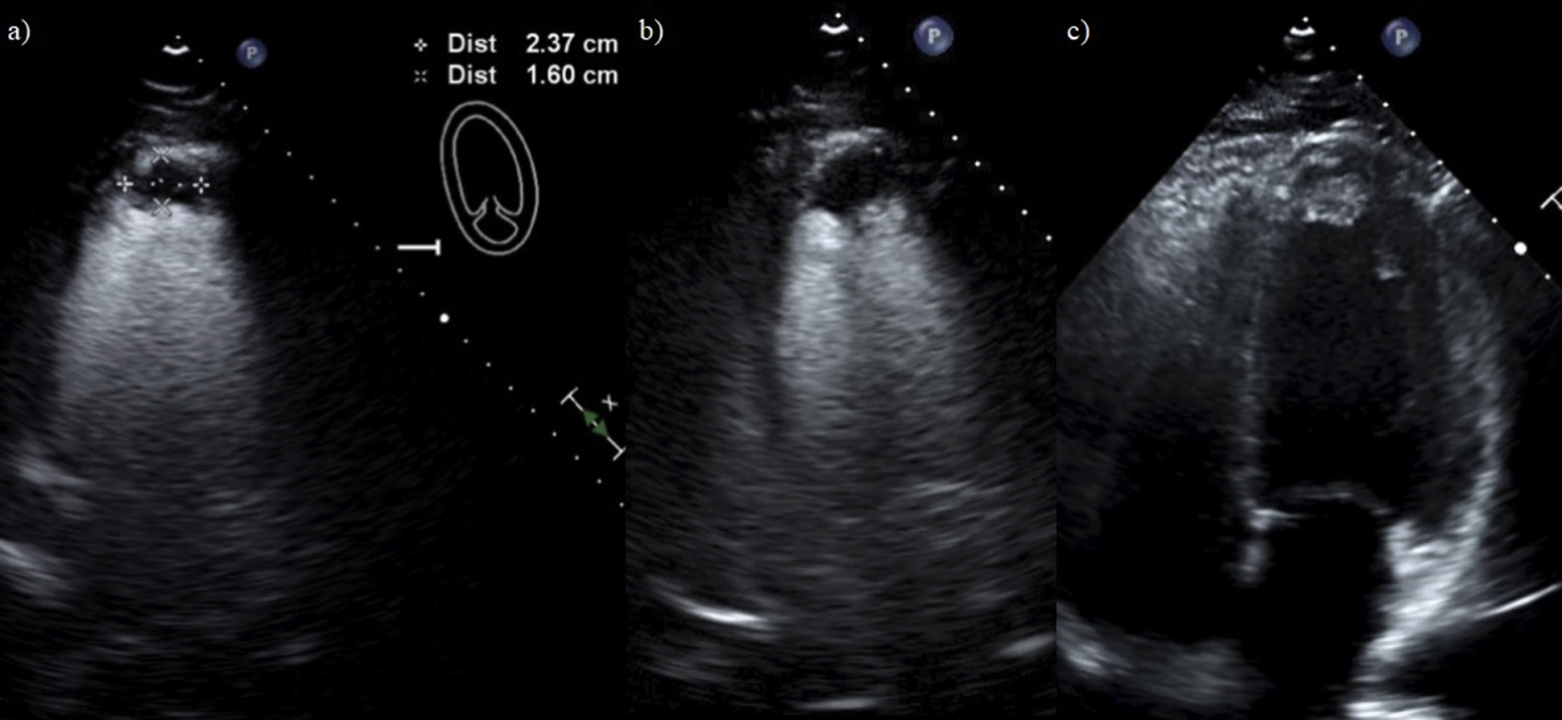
Five-month follow-up transthoracic echocardiogram with and without perflutren lipid microsphere contrast. (A) Perflutren lipid microsphere contrast-enhanced apical two-chamber view of the LV apical mass on follow-up imaging 5 months from the initial images demonstrating reduced size of the mass. (B) Perflutren lipid microsphere contrast-enhanced apical four-chamber view of the LV apical mass on follow-up imaging demonstrating reduced size of the mass. (C) Apical four-chamber noncontrast grayscale view of the LV apical mass on follow-up imaging demonstrating reduced size of the mass.
